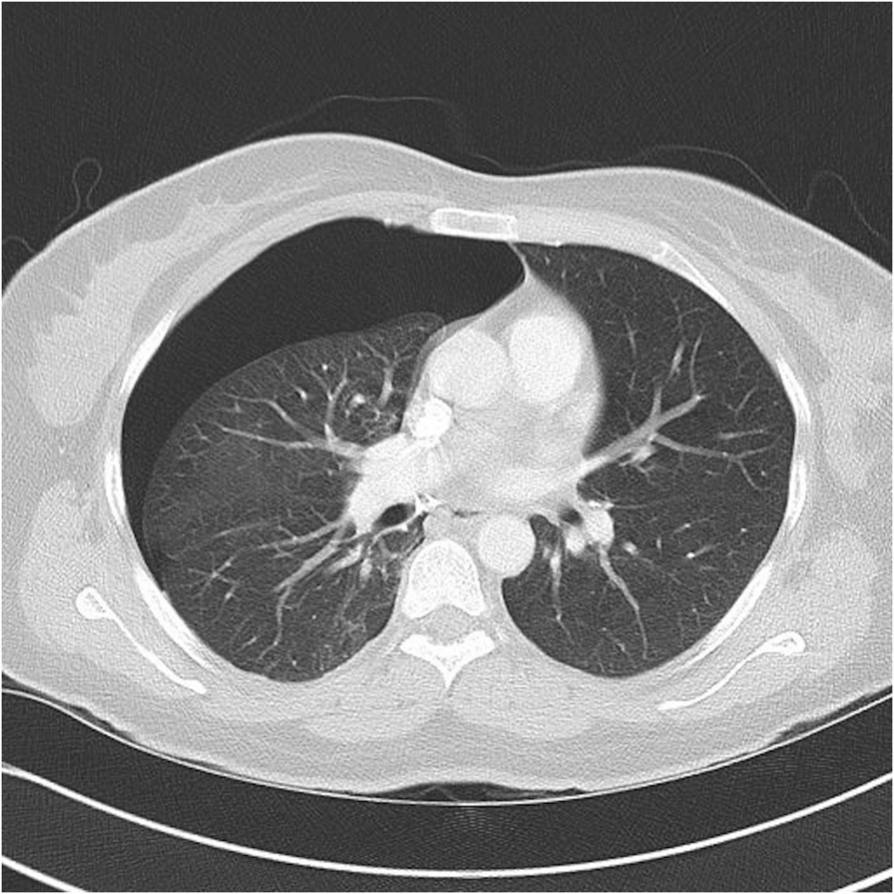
High resolution computed tomography scan of the chest. Large mantle pneumothorax at the level of the right middle lobe, observed 3 days after hysterectomy.
